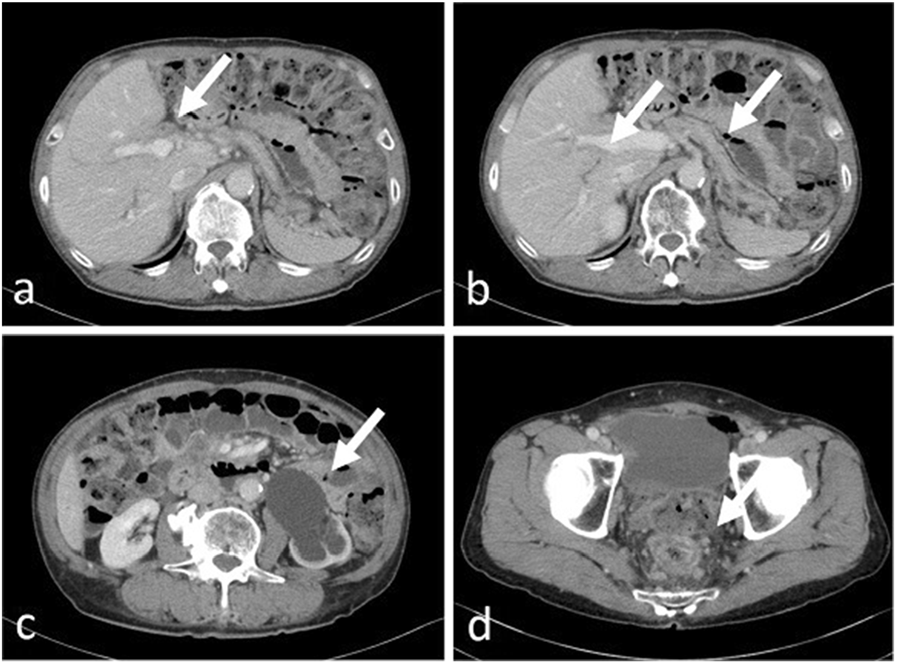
CT findings. Thickening of the hilar bile duct (a: *arrow*) and dilatation of the intrahepatic bile duct (b: *arrow*) were shown. The pancreas was not enlarged (b: *arrow*). Dilatation of the left renal pelvis (c: *arrow*) and thickening of the rectal wall (d: *arrow*) were shown
